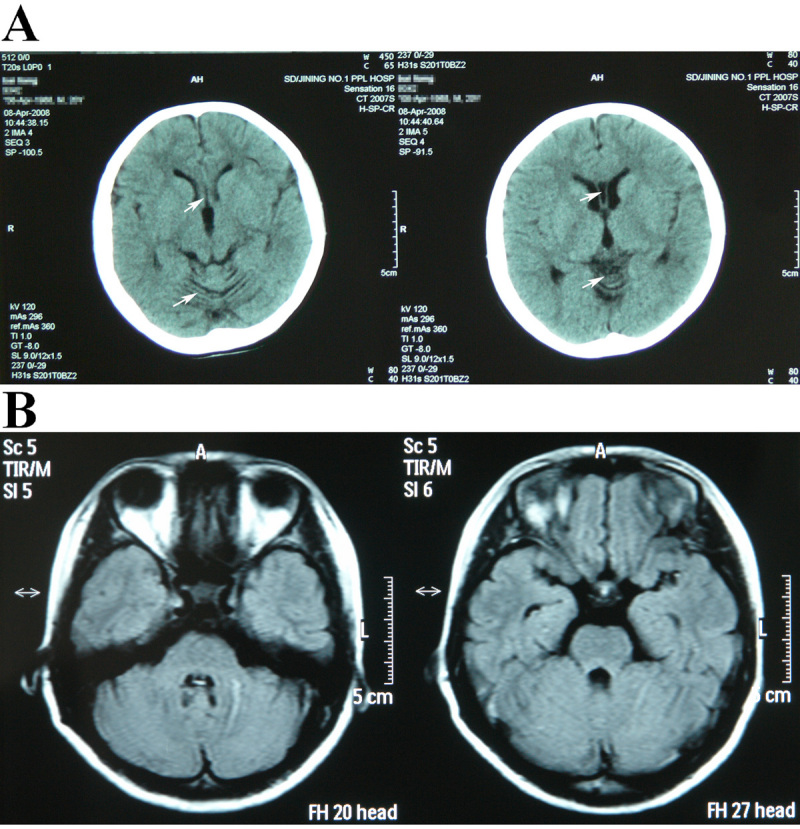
The CT scan and MRI scan results. A: CT scan of the proband’s brain; these images show cerebellar atrophy with widened and deepened sulci and permit observation of the fifth ventricle. Arrows show cerebellar atrophy is characterized by narrowed lobes and widened folds of cerebellar hemisphere and vermis; and the evolution of the cavity of septum pellucidum. B: MRI scan of carrier III:6’s brain; no abnormality was observed.
